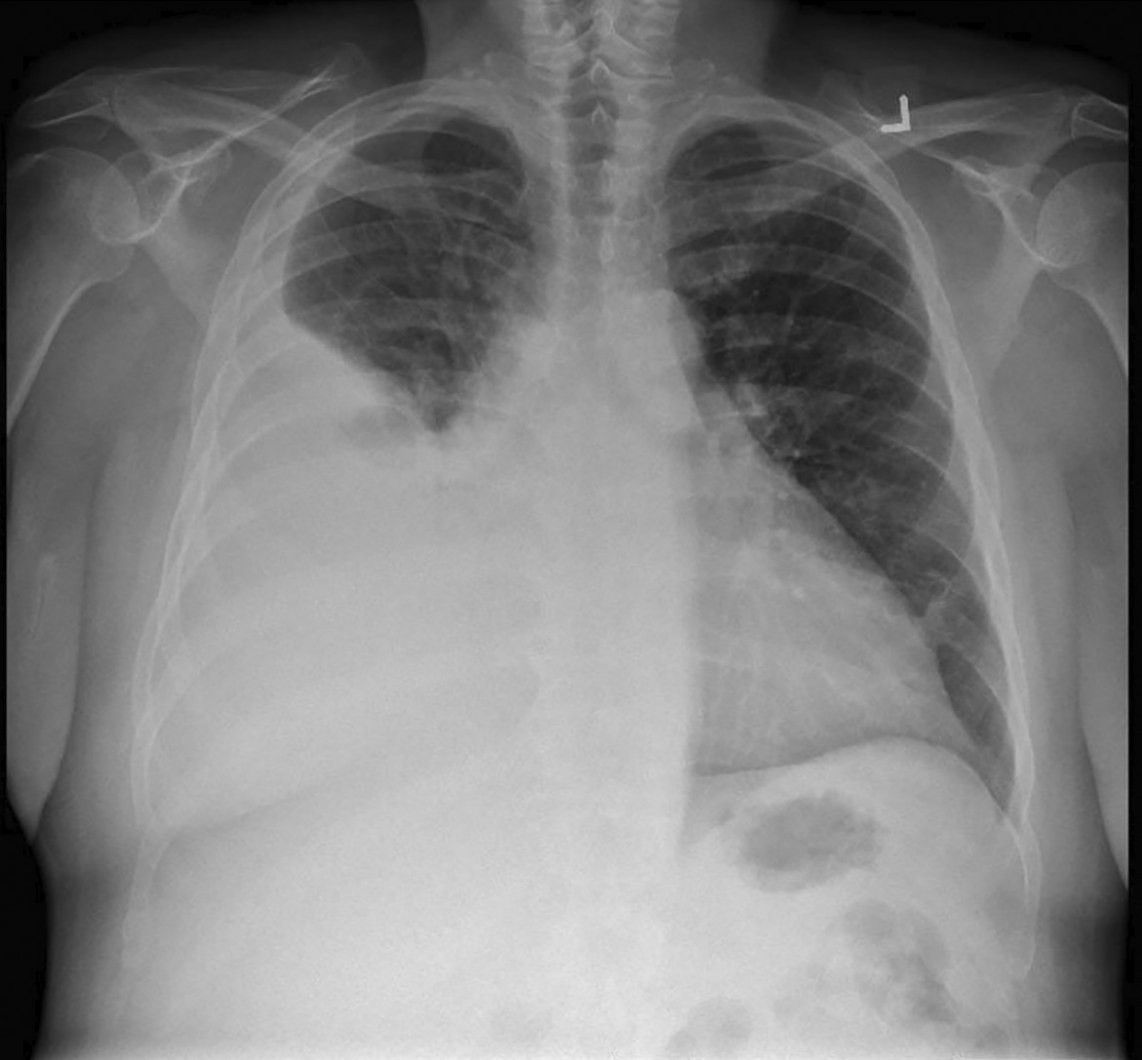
Initial chest radiograph demonstrating large right pleural effusion.
